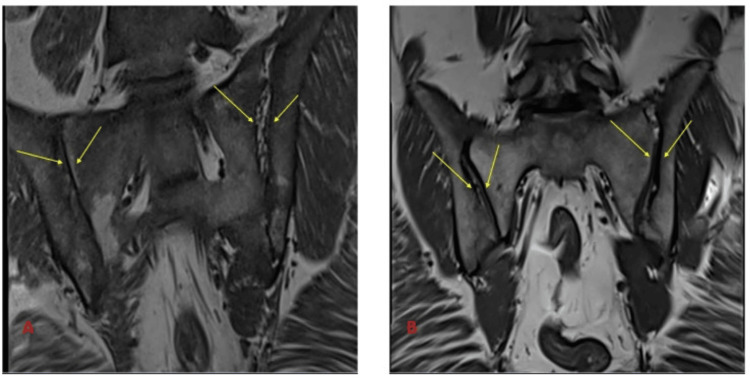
MRI T2-weighted image of sacroiliac joints before and after treatment (A) The baseline MRI T2-weighted image highlights significant sacroiliitis with evident joint inflammation, effusion, and structural abnormalities. These findings are consistent with active disease and inflammatory damage prior to treatment initiation. (B) The follow-up MRI T2-weighted image taken after 12 months of infliximab therapy demonstrates a marked resolution of inflammation and significant improvement in joint integrity, with no new erosive changes observed. MRI: magnetic resonance imaging
